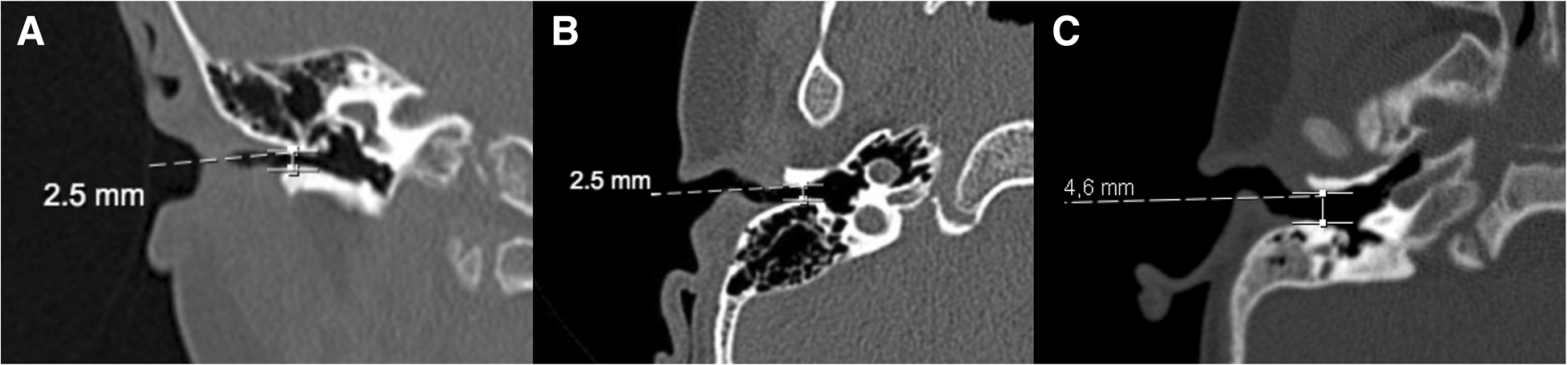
Coronal (a) and axial (b) reconstruction of a temporal bone CT of a 2-year-old boy with Down syndrome, at the level of the mesotympanum, depicting a right stenotic external auditory canal (less than 4 mm in diameter as defined by Cole and Jahrsdoerfer, 2009). Axial reconstruction (c) of a temporal bone CT of a normal 2-year-old boy for comparison. Measurement is done in the bony component of the external auditory canal at the most stenotic point
